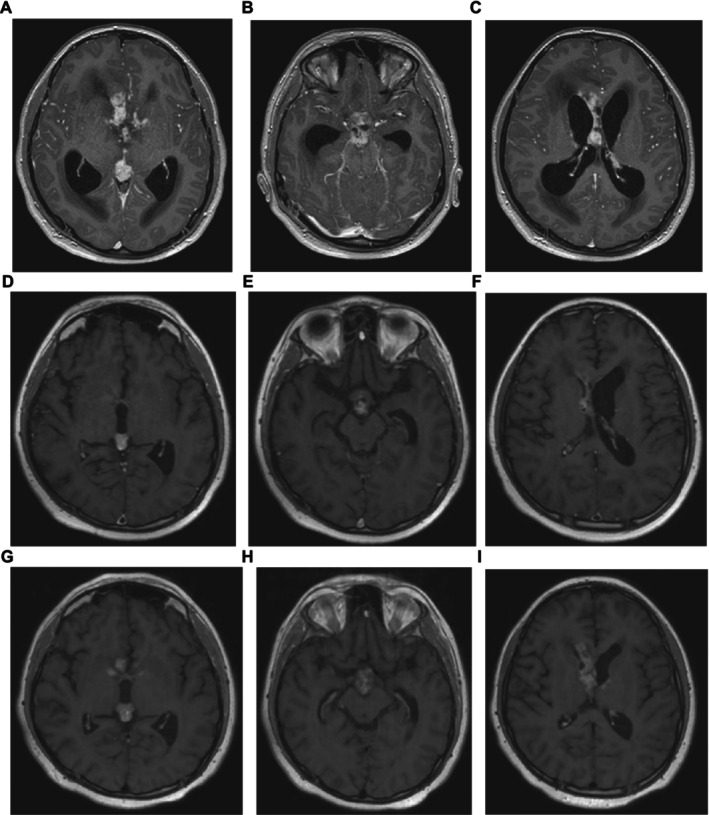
Representative radiology of the metastatic bifocal germinoma case over early time points from diagnosis. Representative axial T1‐weighted post‐contrast MRI head images. (A–C) At diagnosis, demonstrating enhancing disease in the (A) pineal, (B) suprasellar and (C) septum pellucidum regions. (D–F) At Day 9 (d9) on dexamethasone, demonstrating reduction in size and enhancement in the (D) pineal, (E) suprasellar and (F) septum pellucidum disease, presumed secondary to dexamethasone driving out the lymphocytic infiltrate from the germinoma lesions. (G–I) At Day 19 (d19) on maintenance hydrocortisone, demonstrating rebound increase in size and enhancement in the (G) pineal, (H) suprasellar and (I) septum pellucidum disease, albeit less than diagnosis, presumed secondary to lymphocytic re‐infiltration of the germinoma lesions upon cessation of dexamethasone.
